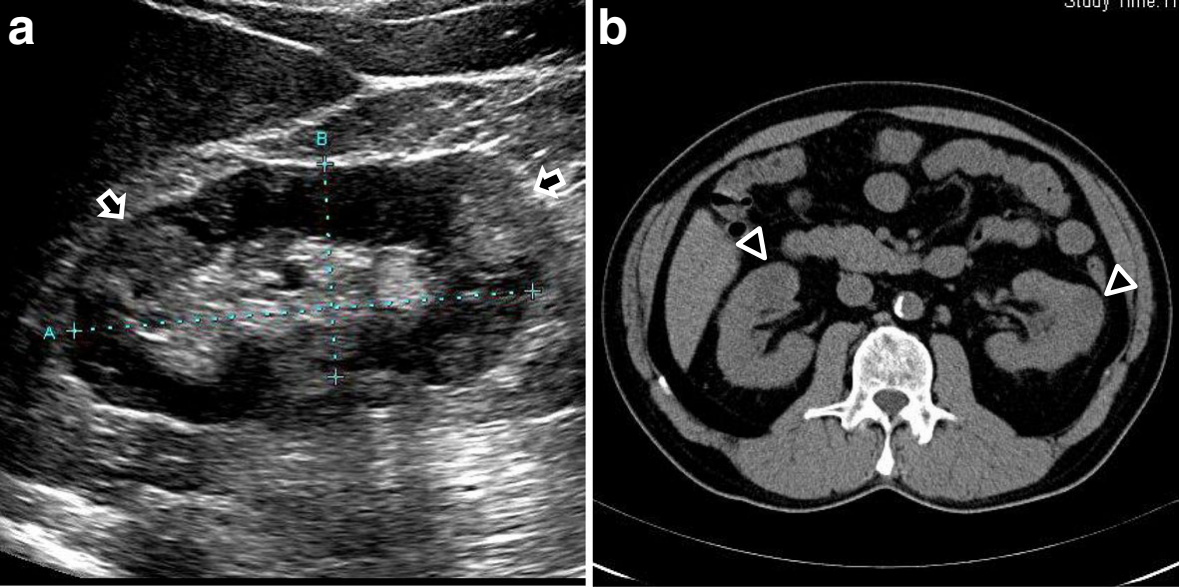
Findings of Echography and computed tomography before treatment. (a) Multiple high echoic lesions (*arrows*) by Echography and (b) mass-like regions (*arrow head*) by computed tomography
